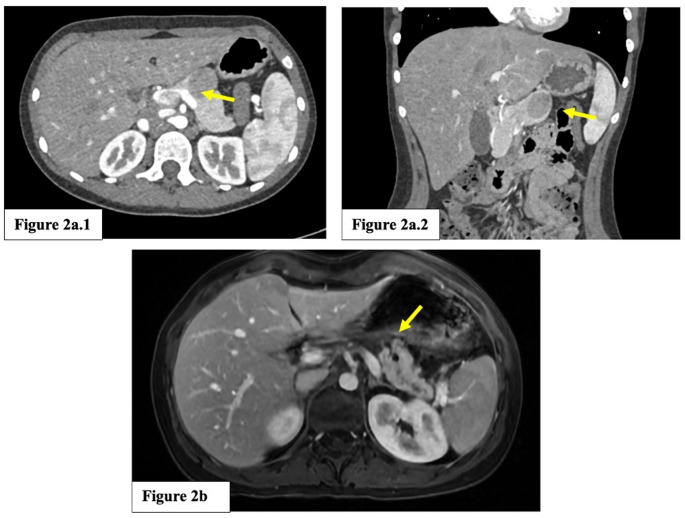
a. Preoperative computed tomography (CT) images. (1) Axial view showing the well-circumscribed lesion (arrow). (2) Coronal view demonstrating the relationship with the surrounding pancreatic parenchyma (arrow). b. Postoperative control MRI. Axial LAVA image shows the Roux-en-Y Pancreaticojejunostomy (arrow) with preserved pancreatic remnant
